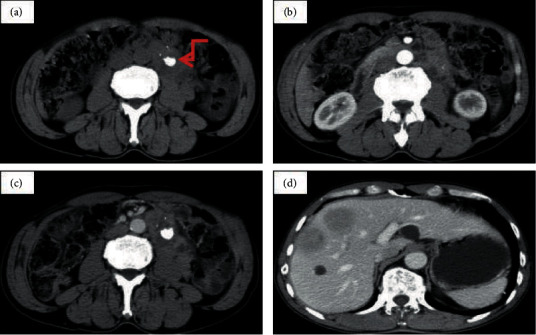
One case of a 67-year-old female with a retroperitoneal neoplasm. (a) Noncontrast CT scan showed an irregular, ill-defined tumour with heterogeneity attenuation and bulky calcification (arrow) was observed. (b, c) Contrast-enhanced scans displayed heterogeneous nonremarkable enhancement. The CT values of the noncontrast, arterial, and venous phases were 43, 79, and 88 HU. The neoplasm extends into the space of the adjacent organs and the left renal artery. (d) Liver multiple metastases occurred 5 years later.
